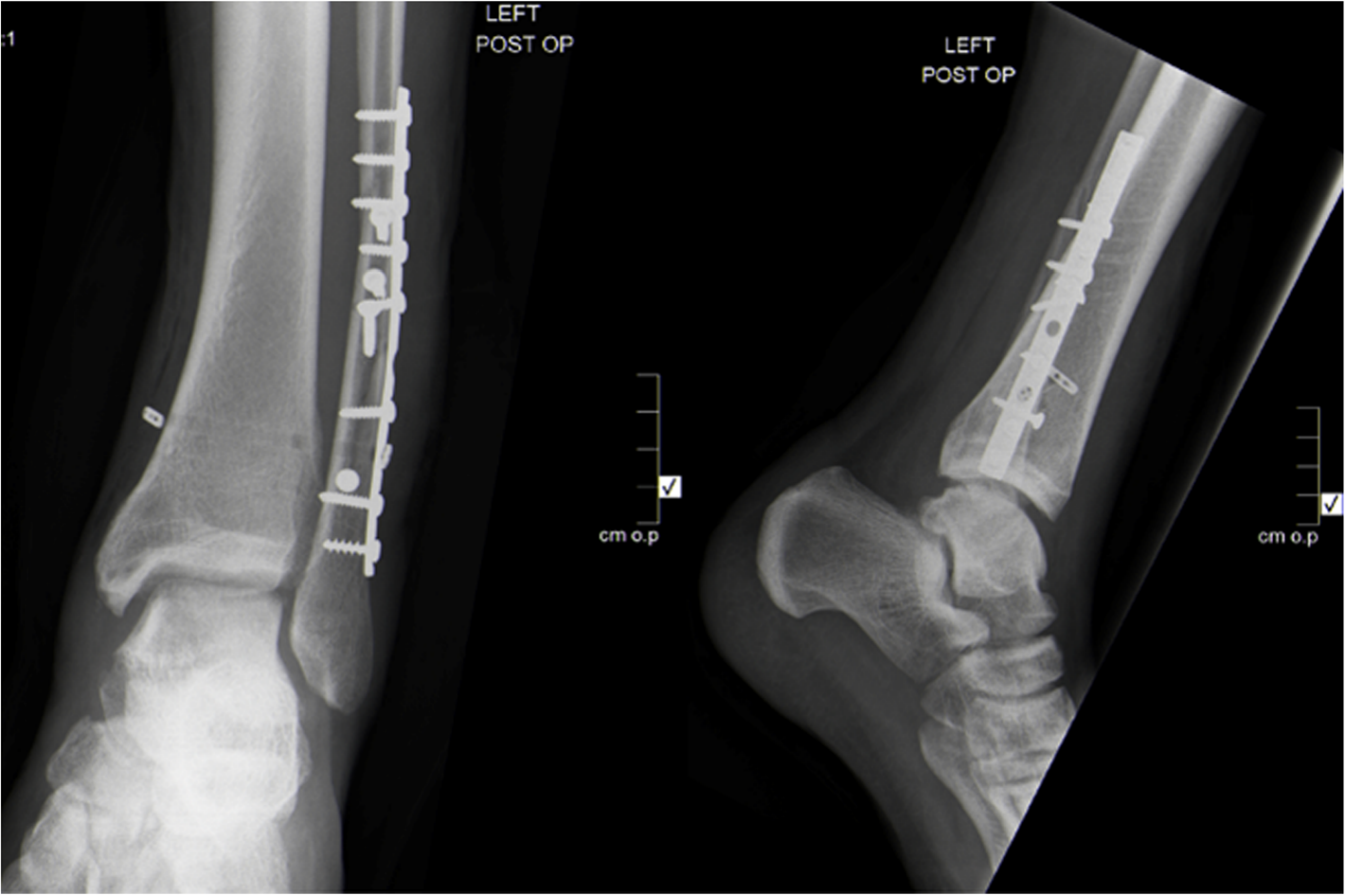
Anteroposterior and lateral plain ankle radiographs after the second operation demonstrating the exchange of the proximal syndesmotic screw with a suture button
